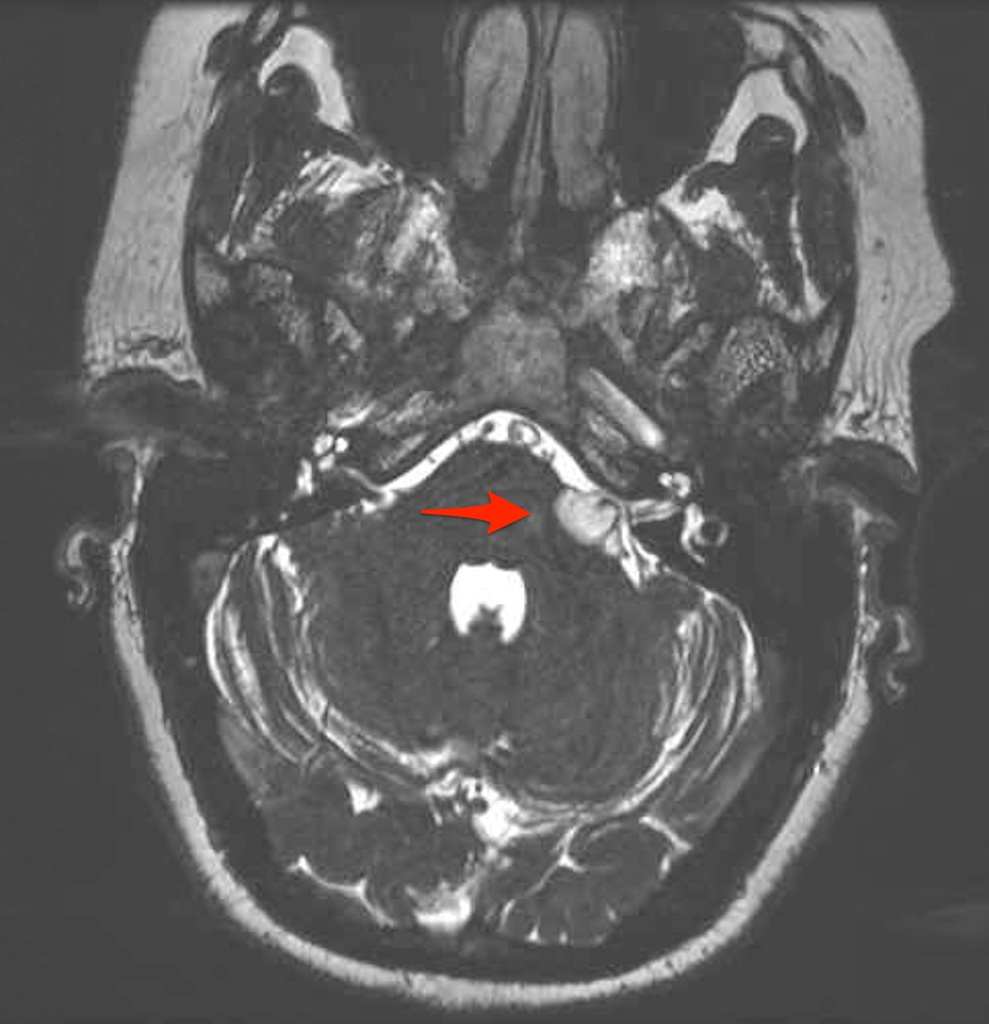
Axial fast imaging employing steady-state acquisition (FIESTA) sequence magnetic resonance imaging (MRI) Image demonstrates a 17-mm hyperintense mass in the left cerebellopontine angle (CPA) with moderate mass effect on brainstem and surrounding structures. Arrow highlights involvement of cranial nerves VII and VIII.
